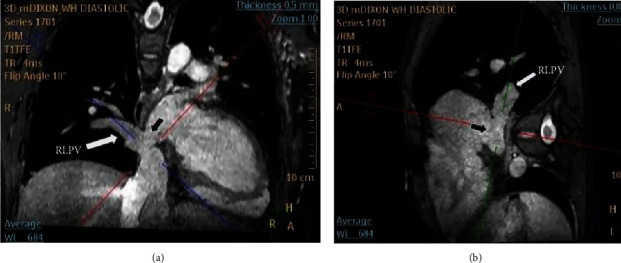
Cardiac magnetic resonance imaging shows a residual sinus venosus defect (black arrow) between the right lower pulmonary vein (RLPV) and inferior vena cava. (a) Coronal view and (b) sagittal view from the left side. Anomalous right lower pulmonary vein drainage can also be observed.
